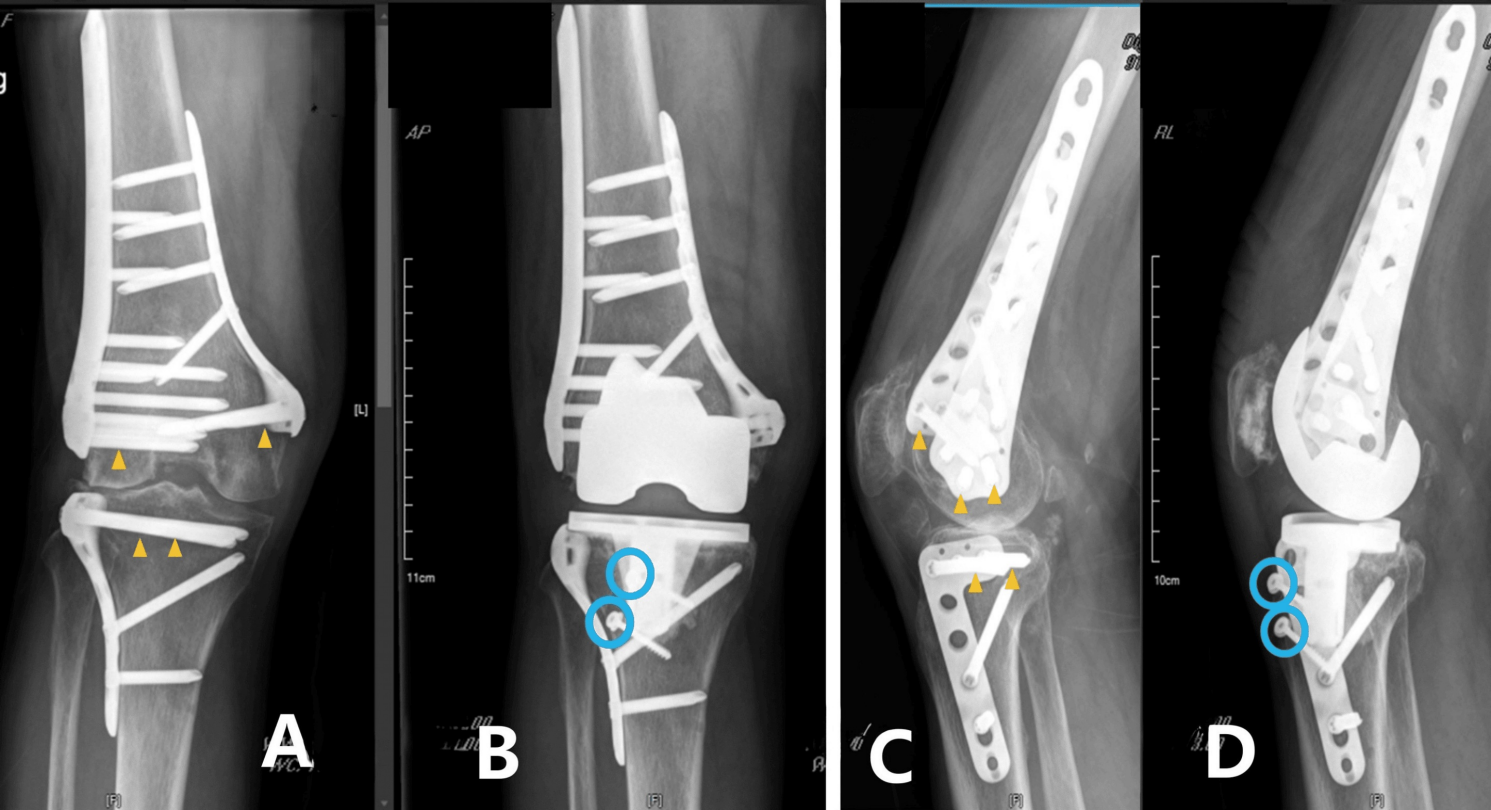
Conversion total knee arthroplasty with minimal periarticular hardware removal (1) A 73-year-old female suffered a floating knee trauma that was treated with locking plates on both sides. Post-traumatic knee arthritis developed two years later with a valgus deformity. During surgery, following CT identification, the blocking screws (yellow arrow) were removed, while two additional screws (hollow blue circles) were added to fix the tibial tubercle osteotomy (TTO) to facilitate exposure to the stiff knee. A, B: radiographic anterior-posterior (AP) view before and after surgery; C, D: lateral projections before and after surgery.
